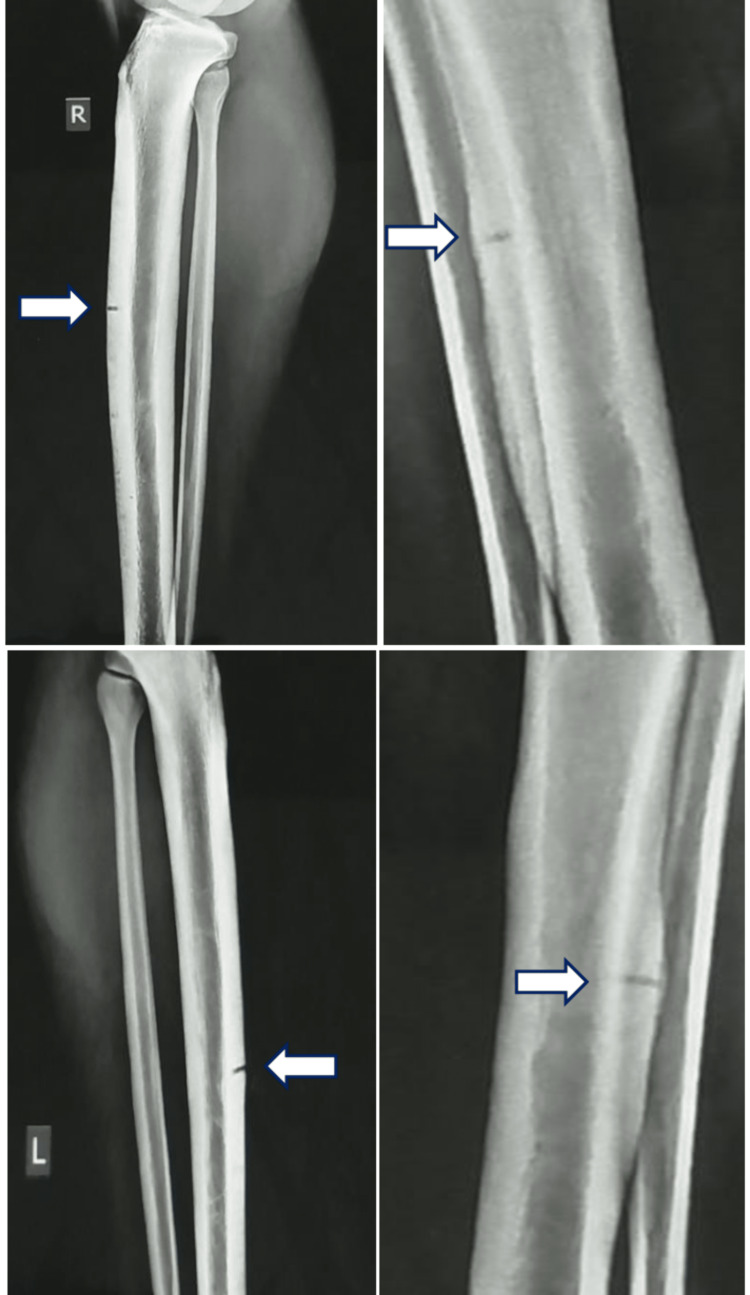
Plain radiographs depicting the synchronous bilateral midshaft stress fractures at the anterolateral cortex of the patient’s tibias
